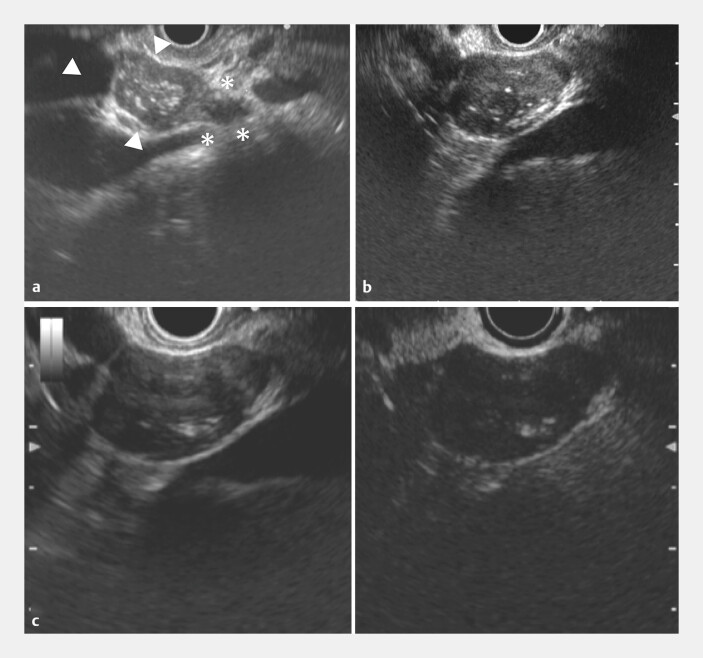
Endoscopic ultrasound (EUS) findings at 6 months after initial presentation.
